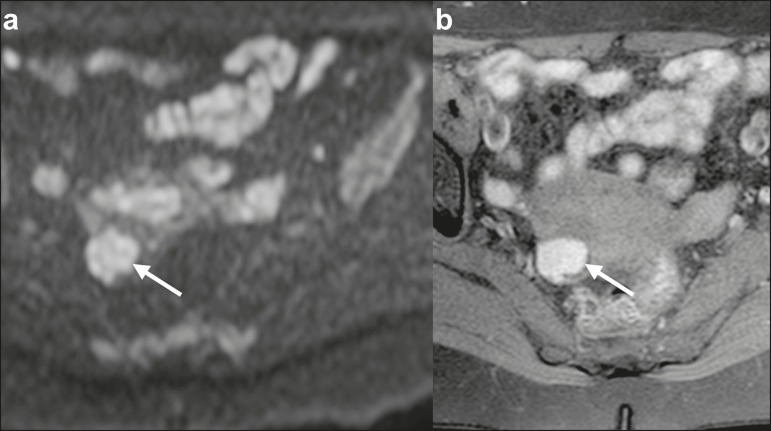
MRI of a female patient with endometrioma. a: Axial DWI at b
